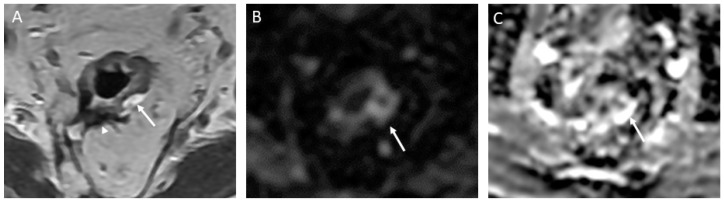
T2 shine-through effect mimicking residual tumor in a 58-year-old man with rectal adenocarcinoma. Axial T2-weighted MR image after the completion of neoadjuvant chemoradiotherapy shows a thick scar along the tumor bed (arrowhead), and mucin extension into the left mesorectal space is noted at the 2:00 to 4:00 position (arrow) (A). On the corresponding axial diffusion-weighted image, there is a notable high signal at the same location as the mucin extension (arrow) (B). An axial apparent diffusion coefficient map (ADC) further confirms the presence of high signal intensity at the location of the mucin extension (indicated by the arrow) (C). This high signal intensity observed on both the diffusion-weighted image and the ADC map indicates the T2 shine-through effect, signifying non-restricted diffusion.
